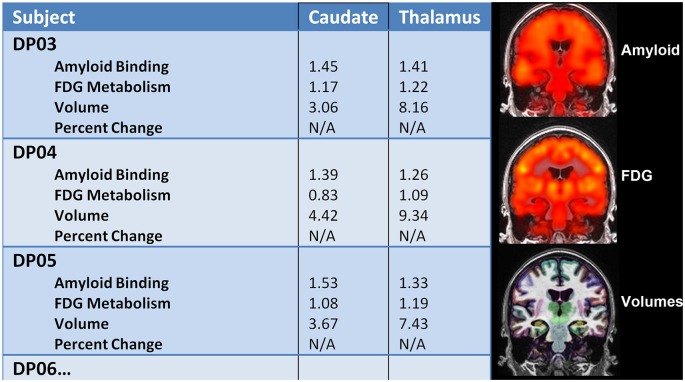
Left: multimodal comparisons can be made in native space within individual subjects longitudinally. Right: Amyloid PET, FDG PET, and volumetric MRI were successfully performed in adults with down syndrome (DS) to capture important structure-function relationships.
